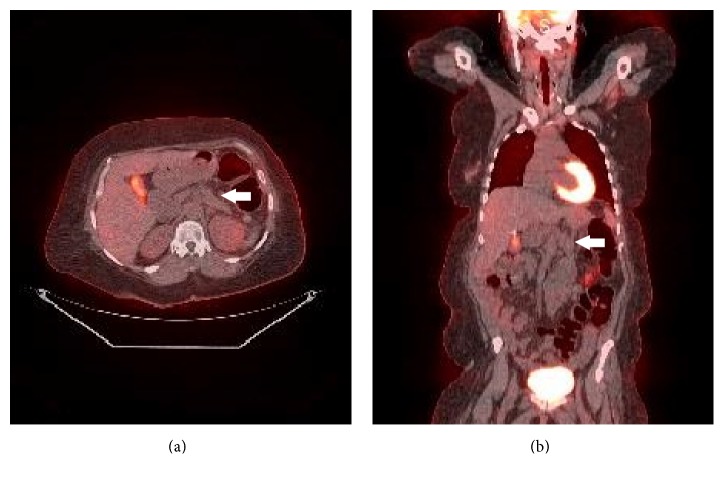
PET-CT ((a) axial, (b) coronal) images showing lack of FDG-avid uptake in pancreas after neoadjuvant chemoradiation.
